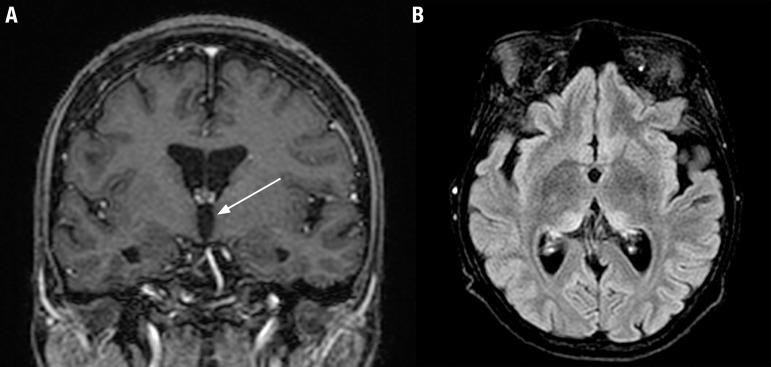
[A] Coronal T1-weighted postcontrast magnetic resonance
